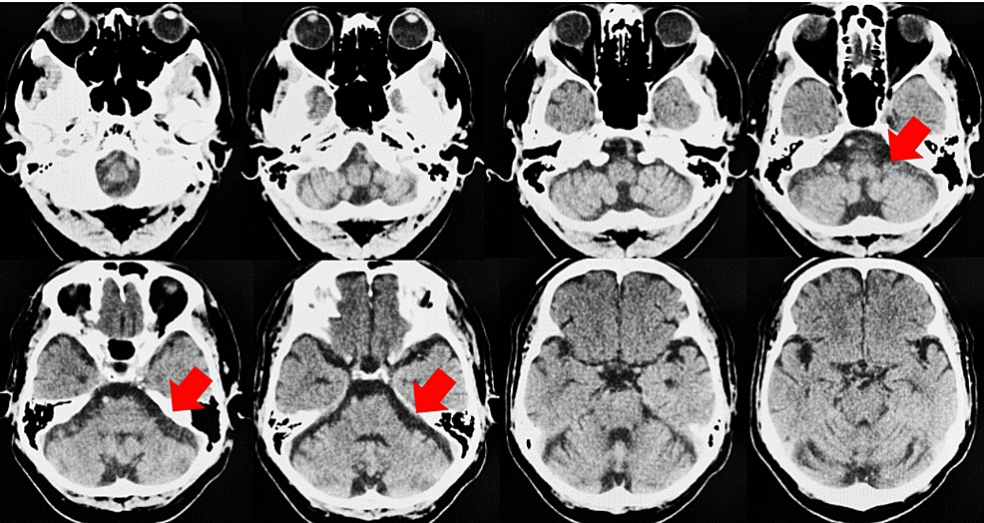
Computed tomography imaging Computed tomography imaging of a 60-year-old male patient with spinocerebellar ataxia type 31 (SCA31), sectioned in the frontal plane. The culmen exhibits prominent atrophy, and the declive, folium, and tuber vermis appear atrophic. Cerebellar atrophy is observed only in the lobulus quadrangularis.
